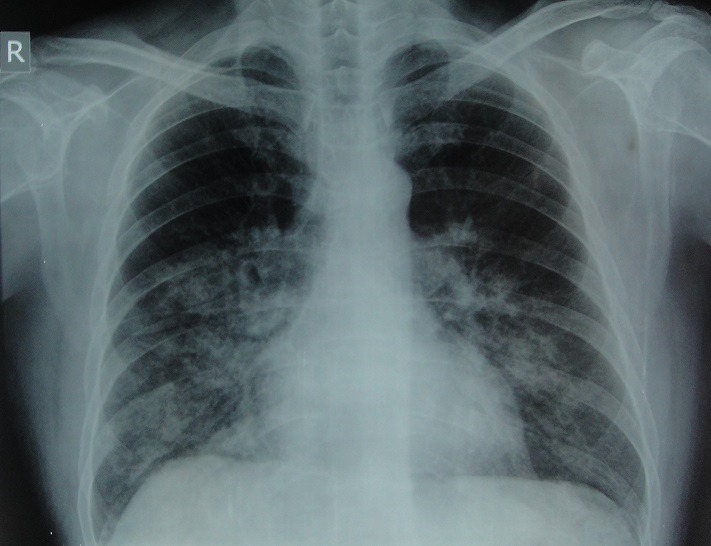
Chest X-ray showing bilateral patchy nodular infiltrate, classically seen in HAPE
